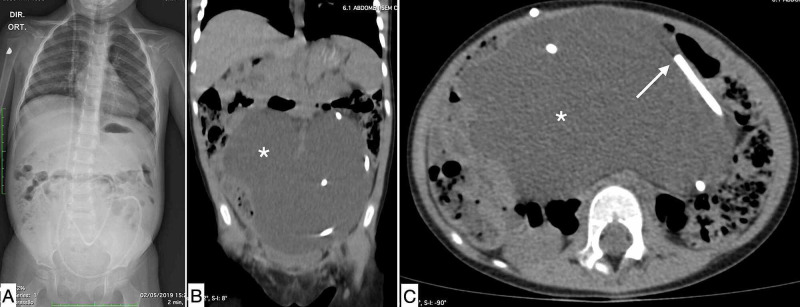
Ascite as an abdominal complication related to VPS. (A) Abdominal X-ray shows its increased size and the distal VPS catheter; (B) coronal view of computed tomography scan of the abdomen shows a homogeneous distribution of peritoneal fluid (*); (C) axial view of computed tomography scan of abdomen depicted the tip of distal catheter (white arrow). VPS: ventriculoperitoneal shunt.
